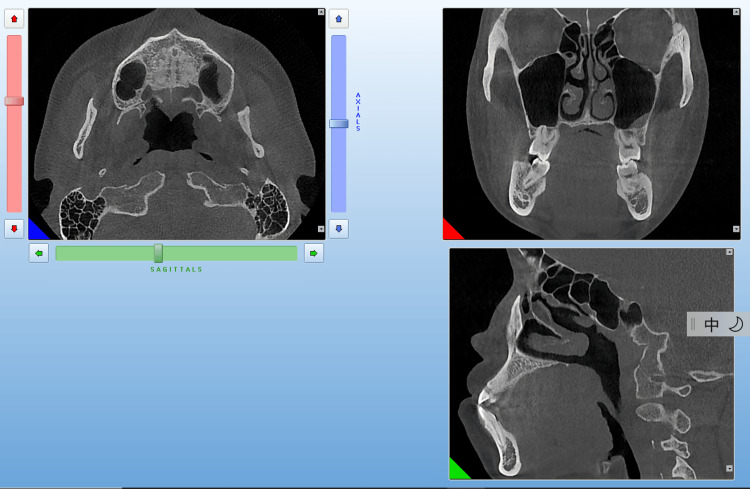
Pretreatment cone beam CT
